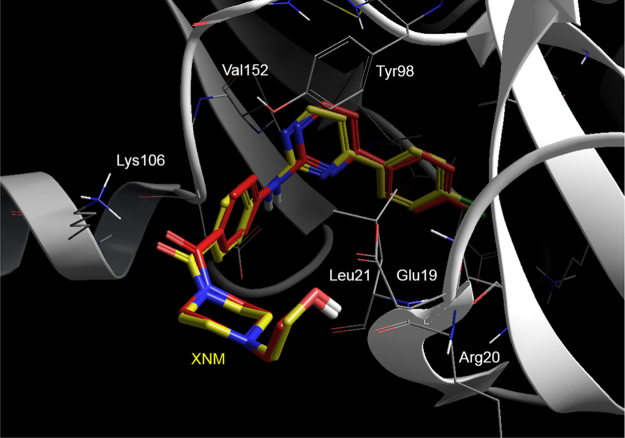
Successful re-docking (red) of XNM back into its original X-ray structures (yellow) by the Standard Precision protocol of Schrodinger's Glide v5.6 used in the docking study.
